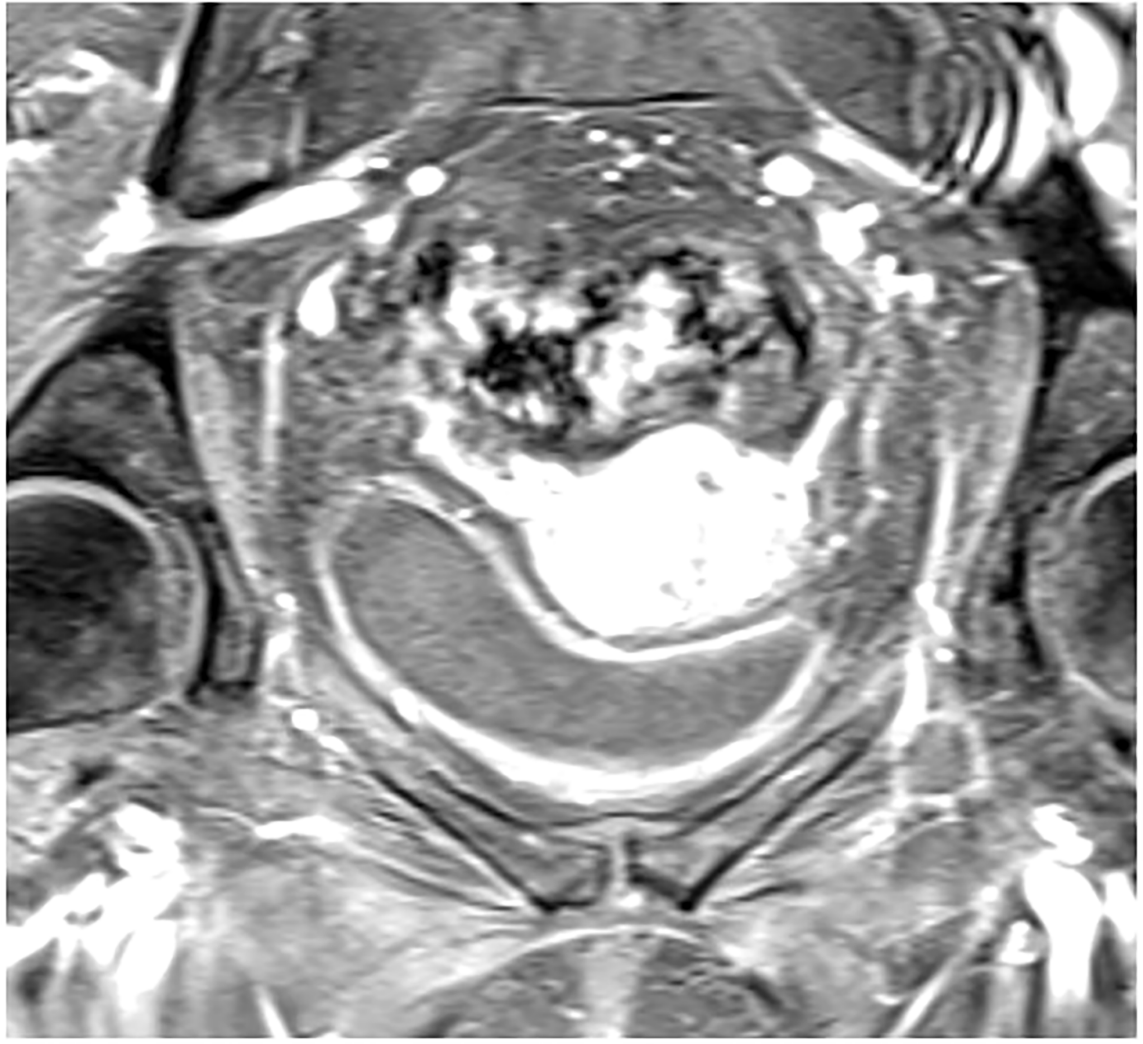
Post contrast dynamic contrast enhanced axial MRI showing increased signal intensity in a patient with FIGO IIIC1, pathologic T2 squamous cell carcinoma of the cervix.
